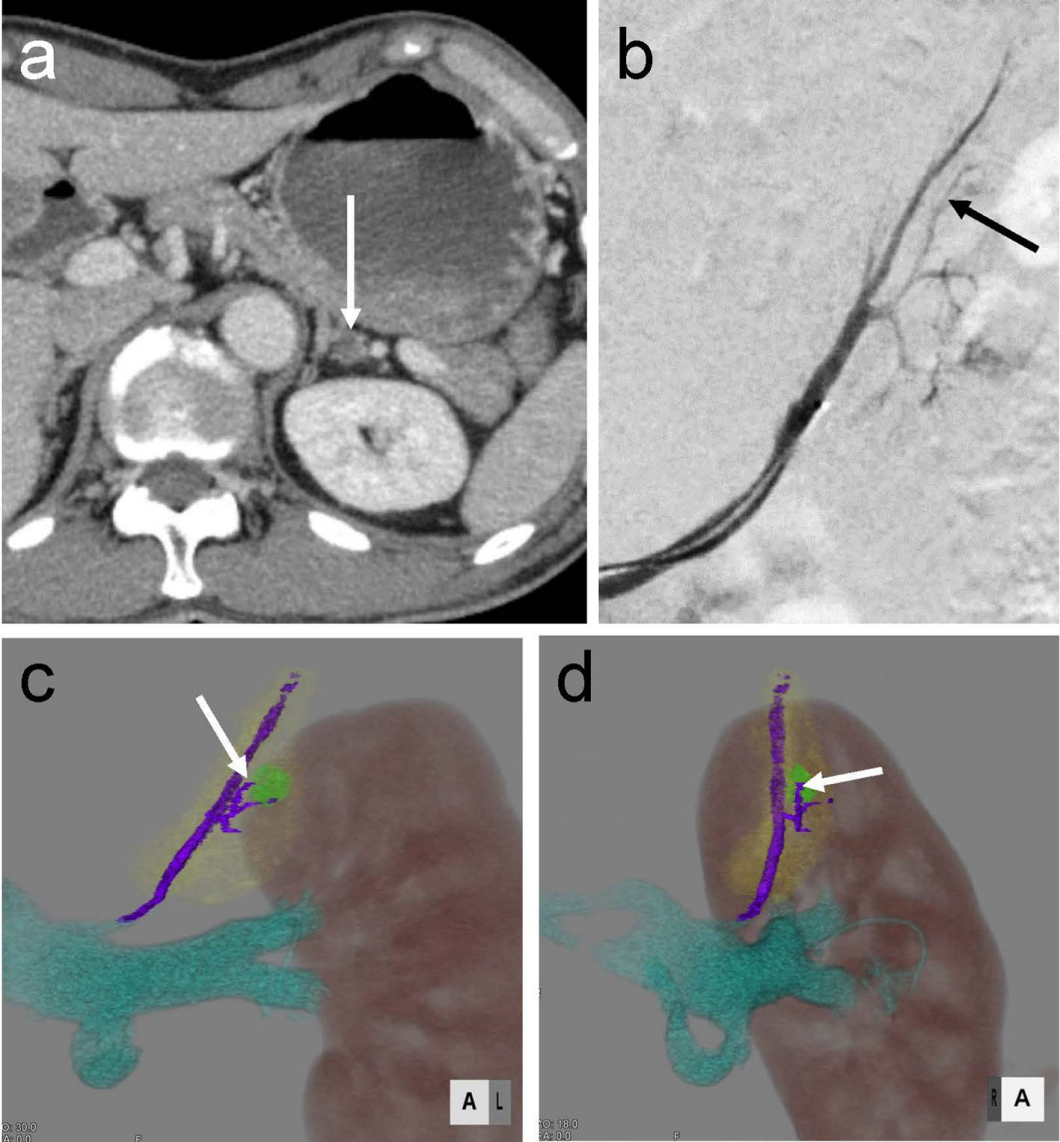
Left adrenal tumor in a 60-year-old man with primary hyperaldosteronism a Dynamic CT section through the left adrenal gland demonstrates a slightly enhancing nodule, 1 cm in diameter (arrow), in the left adrenal gland. b Digital subtraction of left adrenal venogram demonstrates the left adrenal and tributary veins. A capsular vein is filled, but the inferior phrenic vein is not due to the valve at its junction with the adrenal vein. A bent section can be confirmed on the central side of the superior lateral tributary vein (arrowhead). c, d Volume rendering (VR) image obtained on dynamic CT showing the main trunk of the adrenal vein and tributary veins (purple), the left renal vein (cyan), an adrenal tumor (green), the left adrenal gland (yellow), and the left kidney (brown). The left anterior and right anterior oblique views on the VR images show a superior lateral tributary vein located adjacent to the adrenal tumor (arrow)
